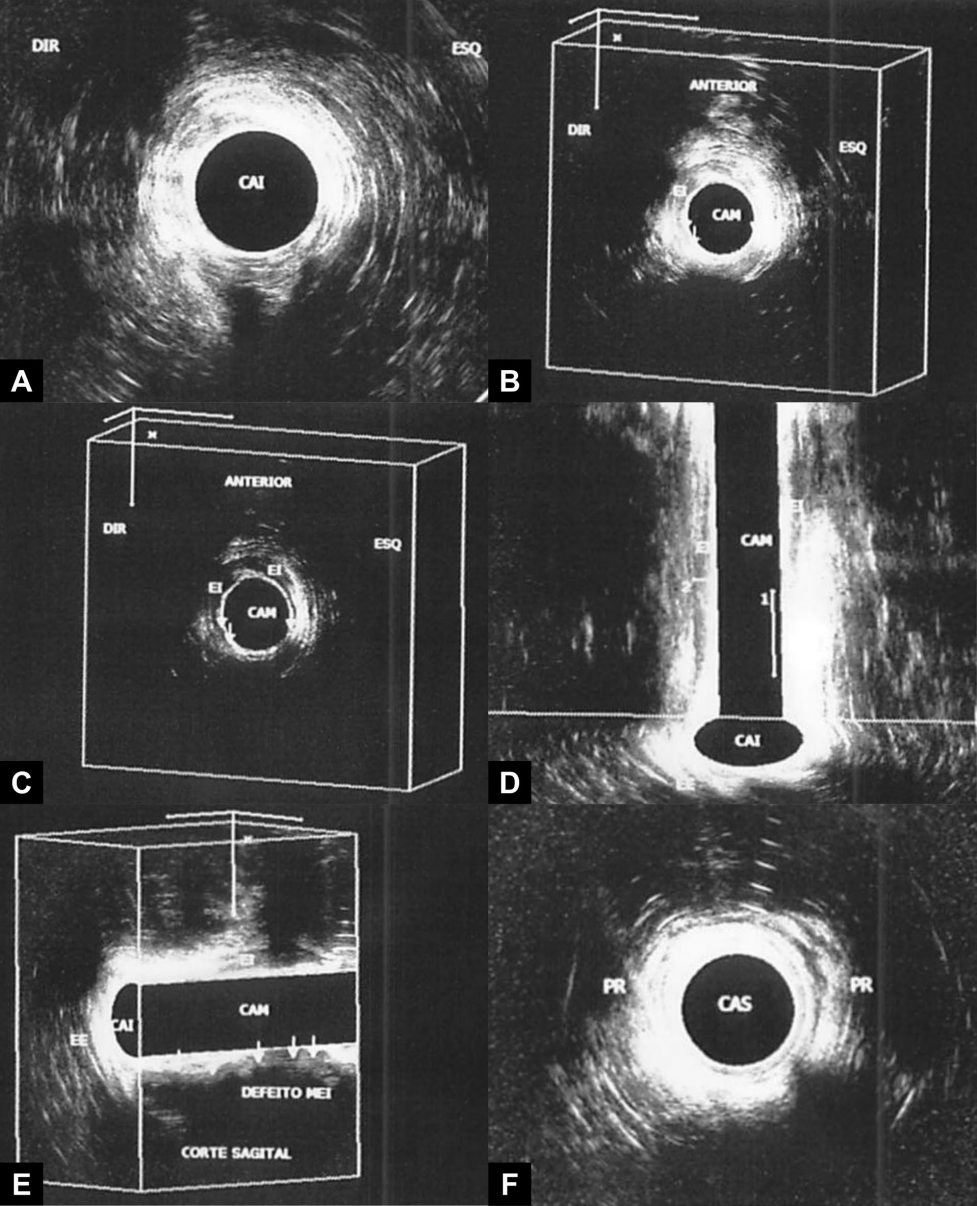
Preoperative 3D endoanal ultrasound showing multiple defects in the internal and external anal sphincters, as well as asymmetry of the puborectalis muscle (Date: 18/01/2024). (A) Upper anal canal: The puborectalis muscle is seen as a hypoechogenic image in the shape of a “V”, with a flaw in the continuity of the muscular cable on the left. (B and C) Middle anal canal: The internal sphincter is seen as a hypoechogenic halo, more evident in the right anterior and anterior quadrants. Two flaws are identified in the other quadrants. The external sphincter muscle also shows interruptions in the right anterolateral and posterior quadrants. (D) Transverse (coronal) section of the three‐dimensional cube: The internal sphincter is visible on the right, with a defect of approximately 14 mm on the left. (E) Sagittal section of the three‐dimensional cube: The internal sphincter is absent in the posterior quadrant, as well as a defect in the external sphincter. (F) Inferior anal canal: The external sphincter muscle shows defects in the right anterolateral and posterior quadrants.
